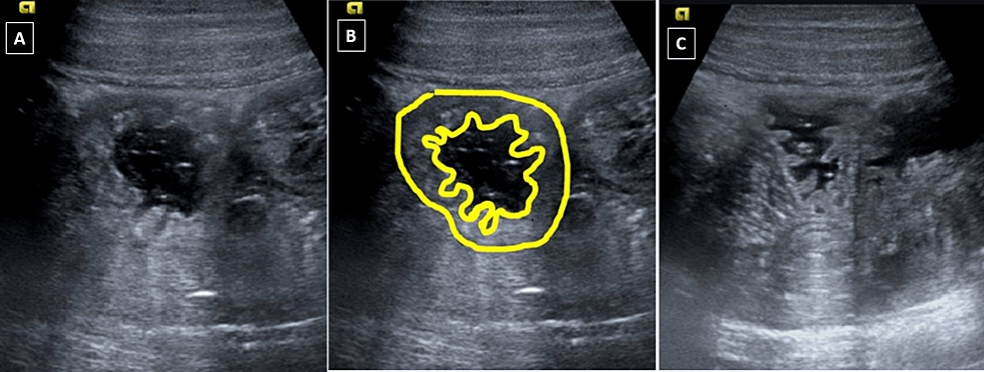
Bowel ultrasonography Figure A shows the bowel wall thickening with thickened mucosal folds. Figure B depicts the same image with the bowel outlined by markings. The inner line represents the mucosal surface, and the outer line represents the serosal surface. Figure C shows the complete resolution of the bowel edema on repeat ultrasonography performed 24 hours later.
